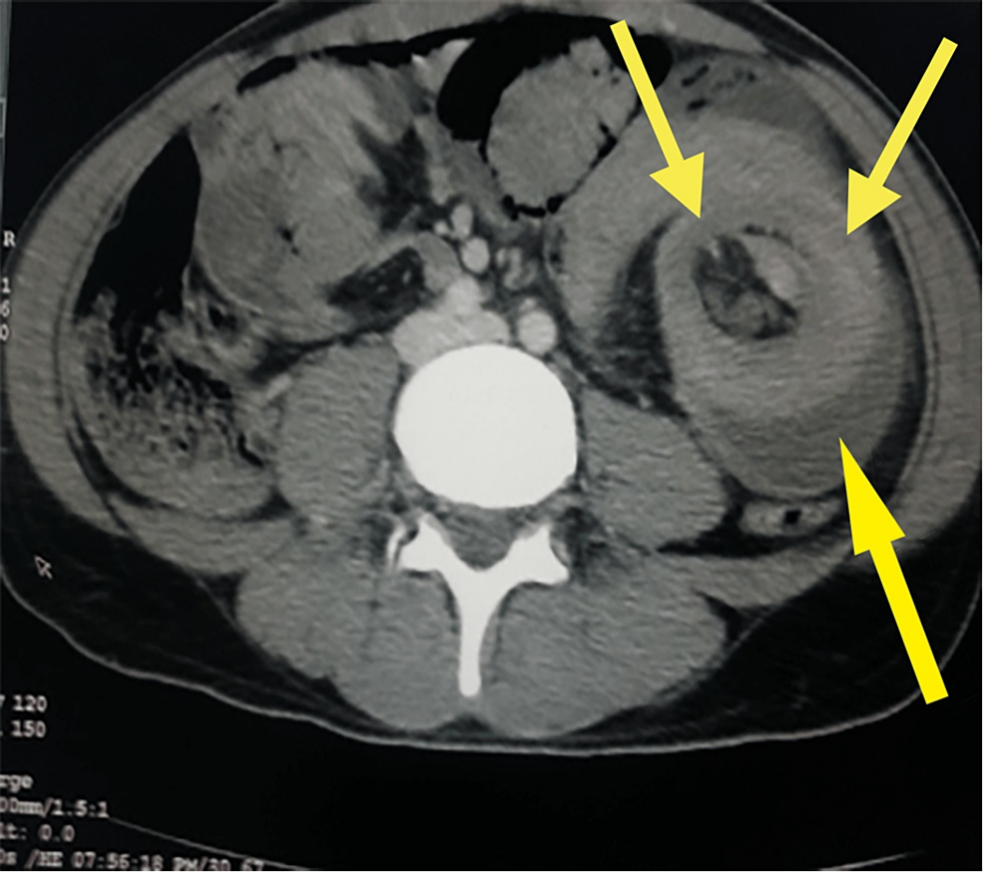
Preoperative computed tomography showing the target sign of intussusception (yellow arrow) which indicates intestinal obstruction. The obstruction was caused by intussusception 2 m from the duodenojejunal junction.
